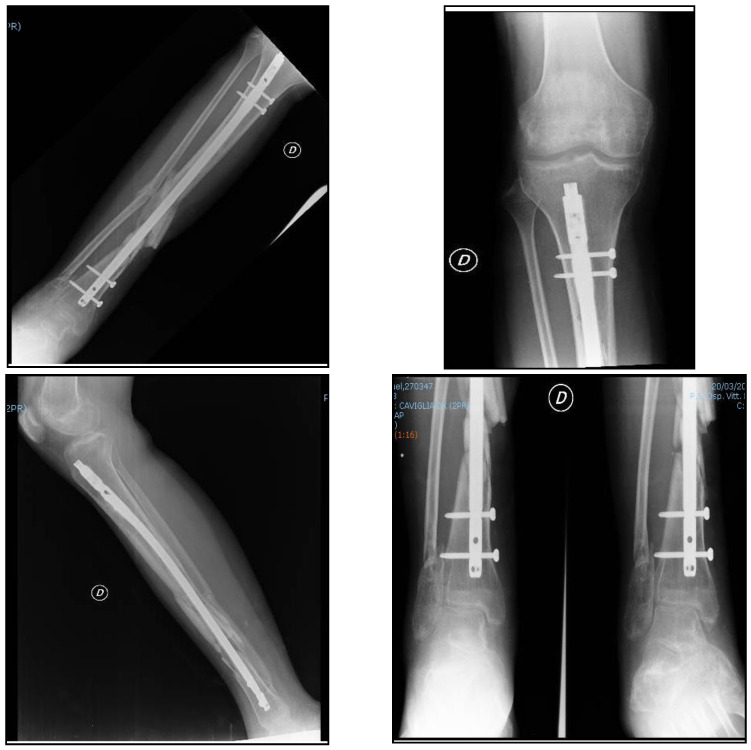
Clinical-radiographic follow up in February 2017.
